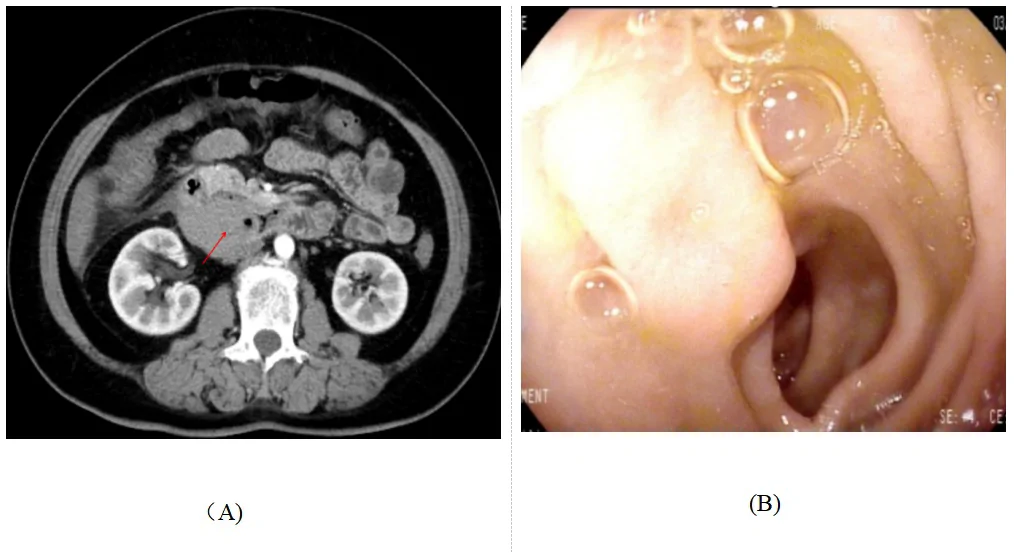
Preoperative imaging and endoscopic findings. (A) Contrast-enhanced computed tomography demonstrated an approximately 6.0 × 3.8 cm relatively well-defined, predominantly solid mass posterior to the pancreatic head. The arterial, portal venous, and delayed phases all showed relatively homogeneous mild enhancement. No progressive enhancement or rapid washout pattern was observed, and there was no apparent heterogeneous enhancement, necrosis, hemorrhage, cystic degeneration, or calcification. The mass occupied the right periduodenal/retroperitoneal space and abutted the inferior vena cava, but its precise organ of origin could not be established. The original radiology report described the lesion as closely related to the duodenum and favored a stromal tumor. (B) Gastroscopy showed mucosal changes in the duodenal bulb. Routine endoscopic mucosal biopsy revealed only heterotopic fundic glands with chronic inflammation and papillary epithelial hyperplasia, which did not account for the deep-seated lesion.
